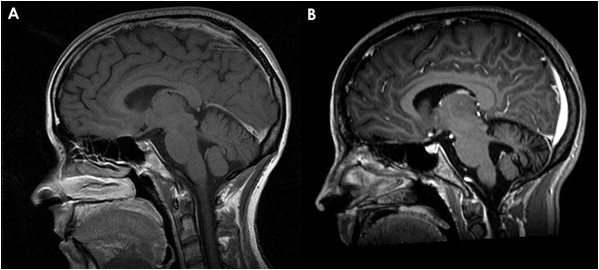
Magnetic resonance imaging of the cerebellum demonstrating marked cerebellar atrophy over the course of disease but no contrast enhancement. (A) T1-weighted sagittal sequence; image obtained at month 3 after onset. (B) Gadolinium-enhanced sagittal T1-weighted sequence; image obtained at month 17. Note the widening of the cerebellar sulci and the fourth ventricle.
